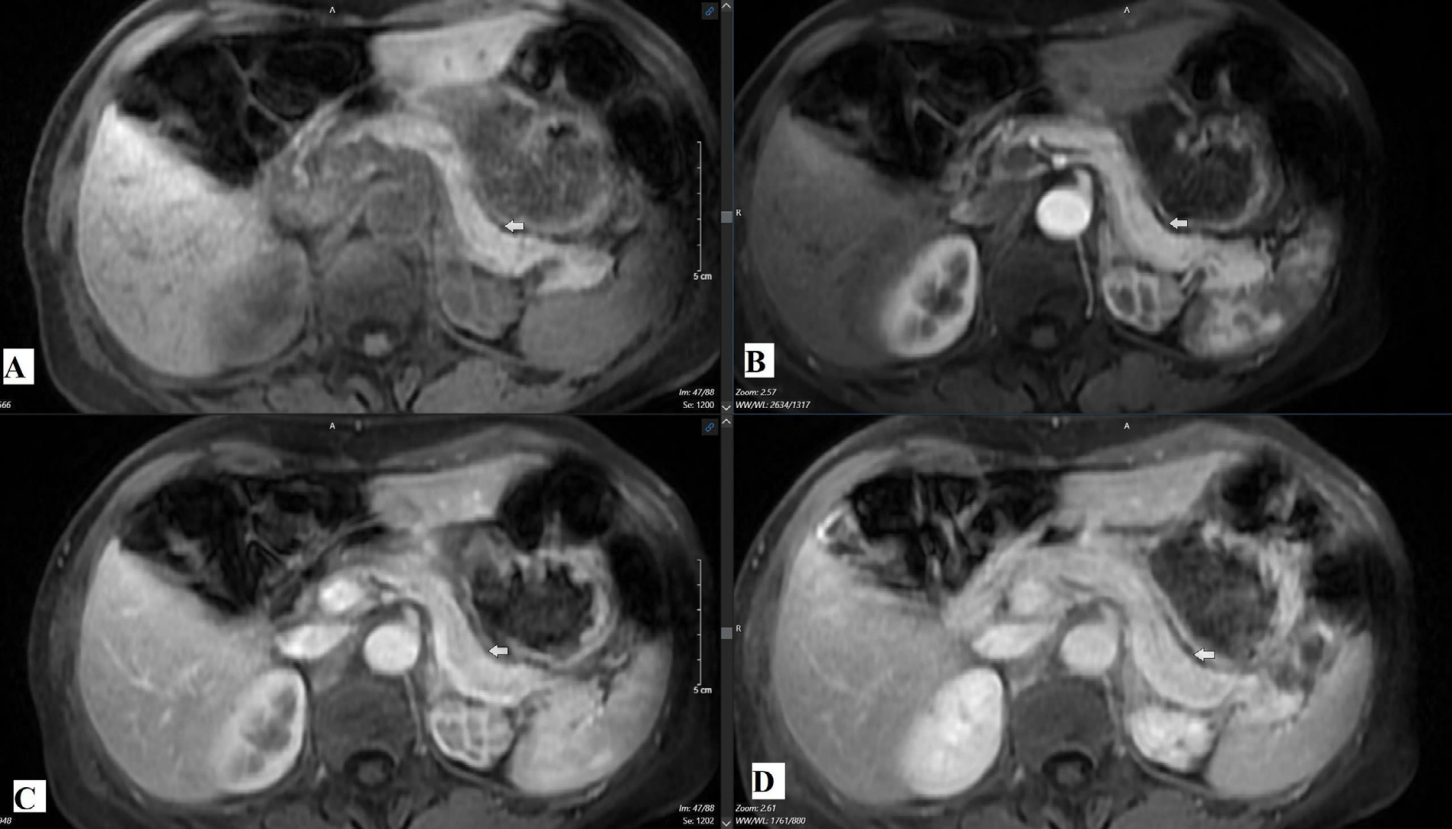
Dynamic contrast-enhanced MRI phases: (A) noncontrast phase, (B) arterial phase, (C) venous phase, and (D) delayed phase. Arrows white in figure A, B, C and D indicate the expected region of the pancreatic tail where no focal lesion was visualized.
